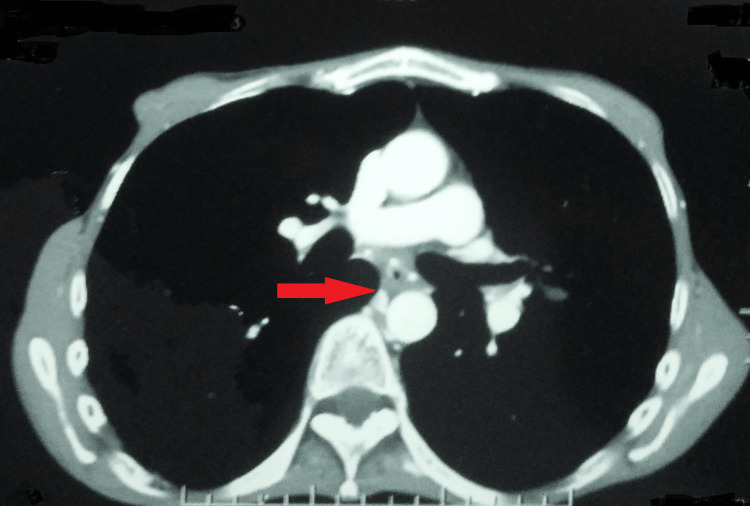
Computed tomography pulmonary angiogram suggestive of hypertrophied and tortuous bronchial artery
